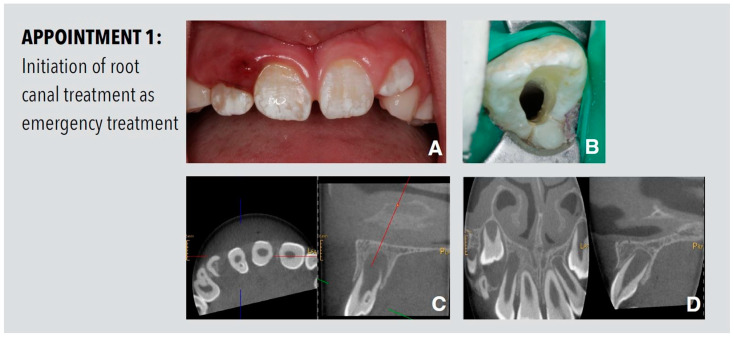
Procedures performed at the first appointment. (A) Preoperative clinical view of the maxillary front teeth showing swelling and redness of the gingiva in proximity to the right lateral incisor. (B) Initial access cavity. (C) Preoperative diagnostic CBCT shows the palatal invagination with partial enamel lining on tooth 12. (D) The sagittal view of the CBCT shows the periapical radiolucency associated with tooth 12 and arrested root development of tooth 12 in comparison to the unaffected tooth 22. The transversal projection of tooth 22 is shown on the right-hand side.
